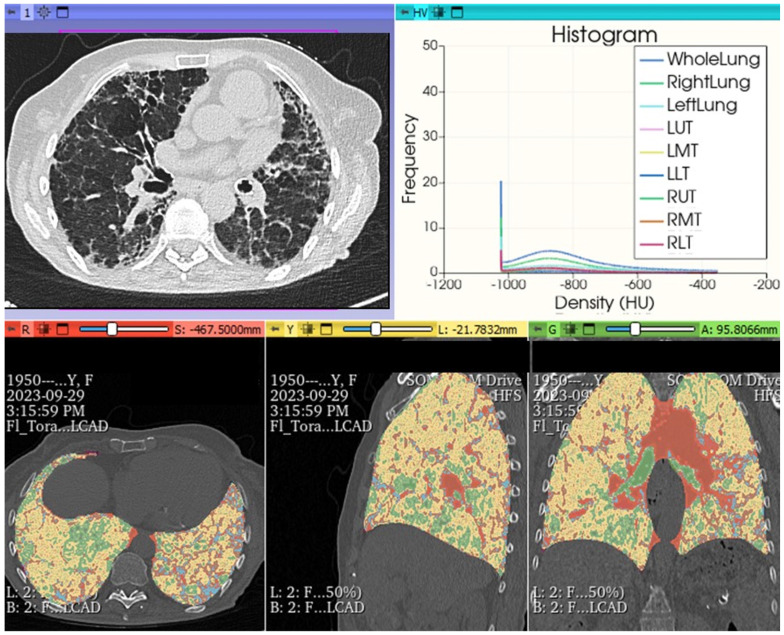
FHP pattern. Chest HRCT: histogram analysis and quantitative reprocessing in MPR on Slicer3D colorimetric scale. AI: radiomics first order features extracted by HRCT after analysis with AI algorithm pyradiomics: whole lungs LAA% −950:13.640; LAA% −910:23.190; HAA% −700:30.560; HAA% −500:16.243; Perc10: −969.000 mean: −719.705; kurtosis: 3.241; skewness: 1.787; ventilation heterogeneity: 0.915; mass 491.189; volume: 1.712; entropy: 2.5032189221693084 energy: 1795731501003.0; variance: 1655.8891640178702; uniformity: 0.18260469191135123.
